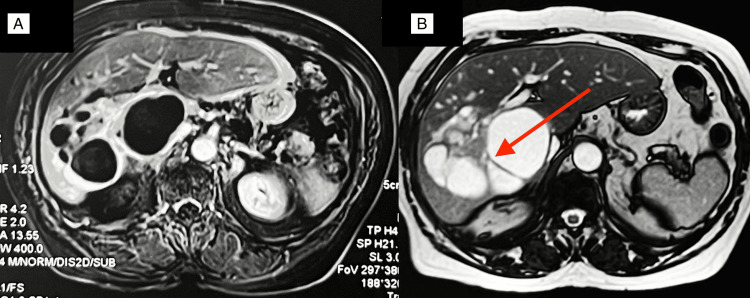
MRI abdomen (A) MRI angiogram in the T1 phase shows a peripherally enhancing hypointense cystic lesion involving segments 6, 7 and 8 of the liver. (B) The lesion is hyperintense in the T2 phase with multiple internal septations (red arrow).
